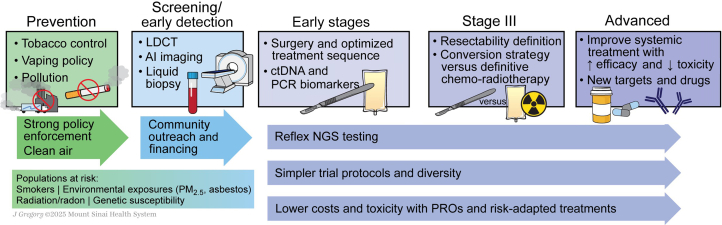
Calls to action from the NYLCF Summit. AI, artificial intelligence; ctDNA, circulating tumor DNA; LDCT, low-dose CT; NGS, next-generation sequencing; NYLCF, New York Lung Cancer Foundation; PROs, patient-reported outcomes.
